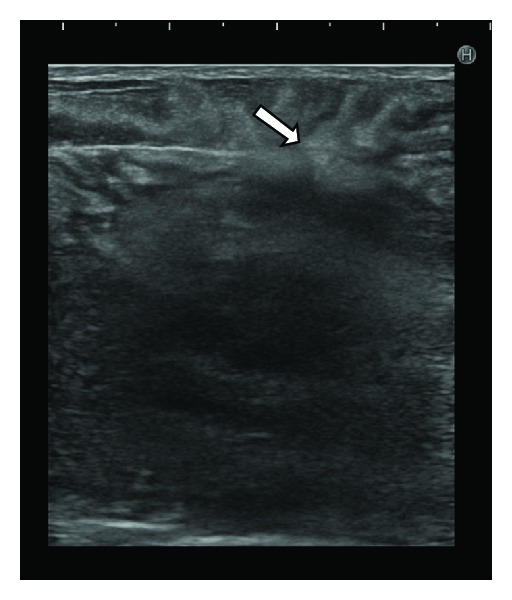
Ultrasound showing a hypoechoic mass with a hyperechoic boundary toward the subcutaneous tissue (arrow).
